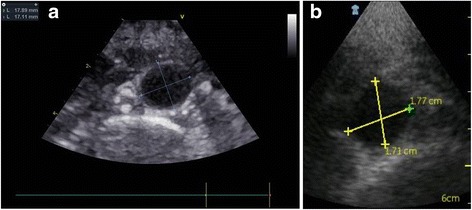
AA visualized in longitudinal and transverse plans from the diaphragm to the bifurcation of the aorta. Antero-posterior and latero-lateral outer diameters were measured in the transverse plane, at the largest portion of infrarenal aorta. The figure shows the good concordance of the two measured diameters between standard echocardiography (panel a) and PSID (panel b): 17.89 mm versus 1.77 cm and 17.11 mm versus 1.71 cm
